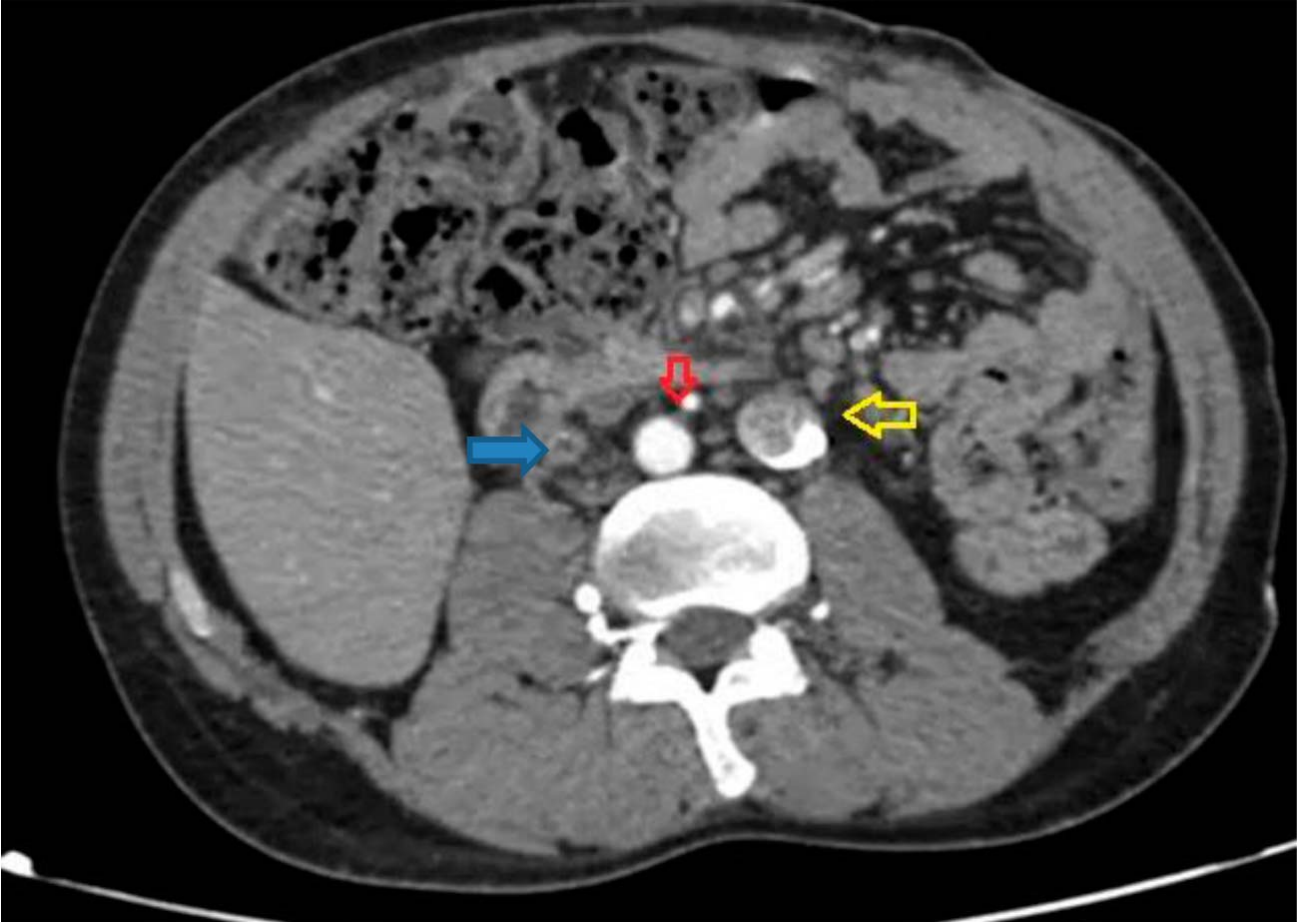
Axial CT scan image demonstrates two venous structures of significant caliber running parallel on both sides of the abdominal aorta, consistent with a duplicated IVC. Both vessels converge at the level of the renal veins, confirming the presence of IVC duplication. The red arrow points to the abdominal aorta, the yellow arrow indicates the left-sided duplicated IVC containing the tunneled catheter, and the blue arrow shows the right-sided IVC, which appears reduced in caliber. CT, computed tomography; IVC, inferior vena cava.
